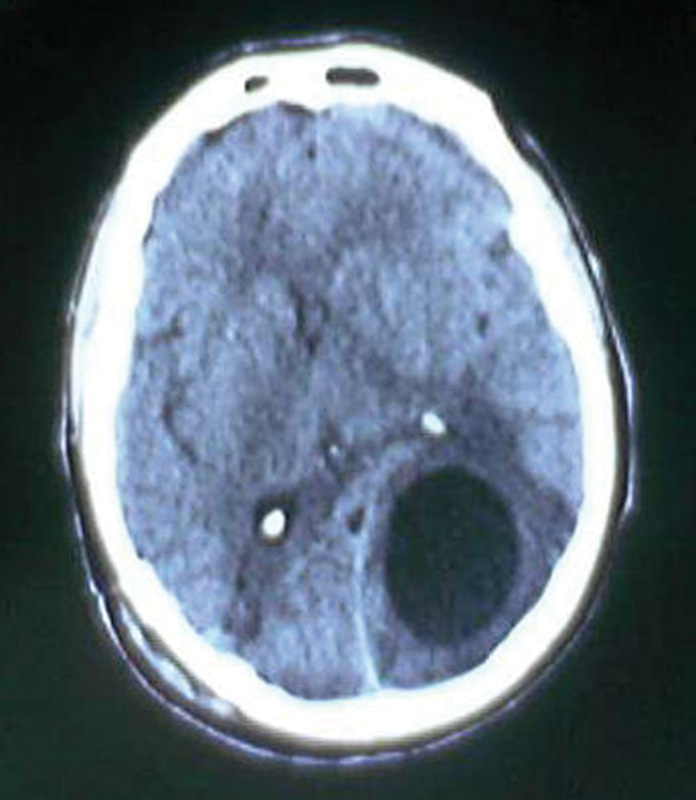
Emergent cerebral computed tomography scan revealing marked expansion of the left occipital lobe cyst with perifocal edema.
